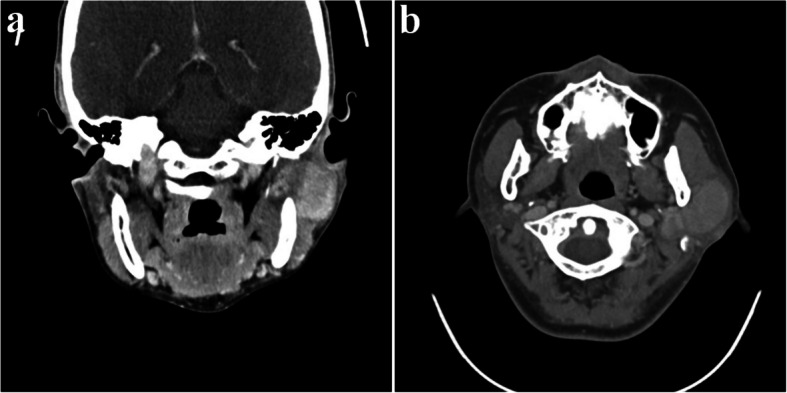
Preoperative CT images (parotid gland area); a well-defined mass in the left parotid gland with homogeneous strong enhancement. a Coronal view, neck extension, b axial view
